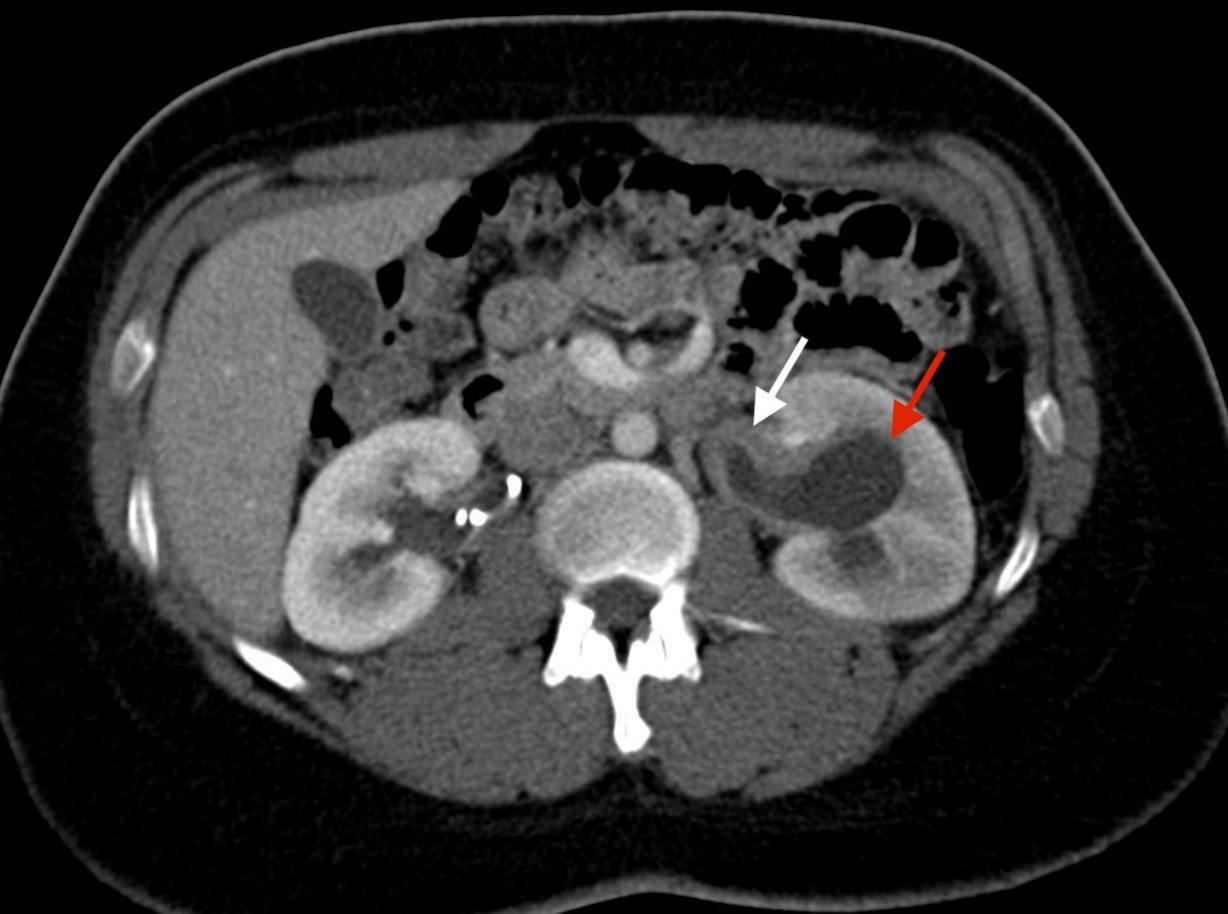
Axial CT image showing thickened left ureter (white arrow) and moderate hydronephrosis of the left kidney (red arrow) CT: computed tomography
